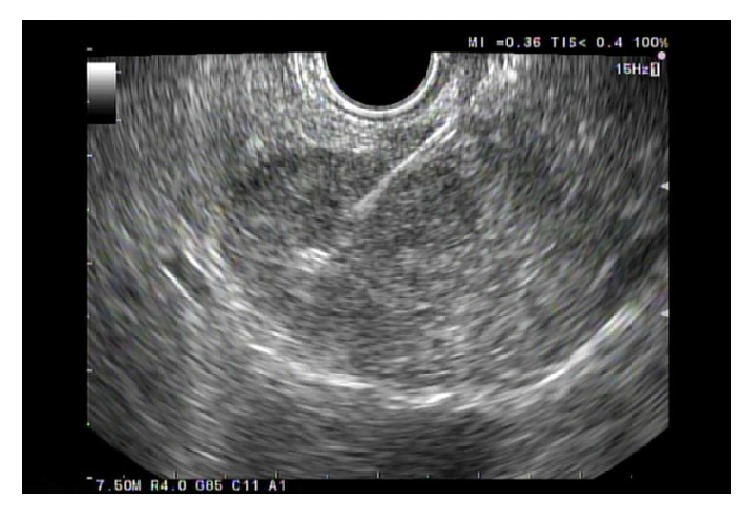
Fine needle aspiration during endoscopic ultrasound.
